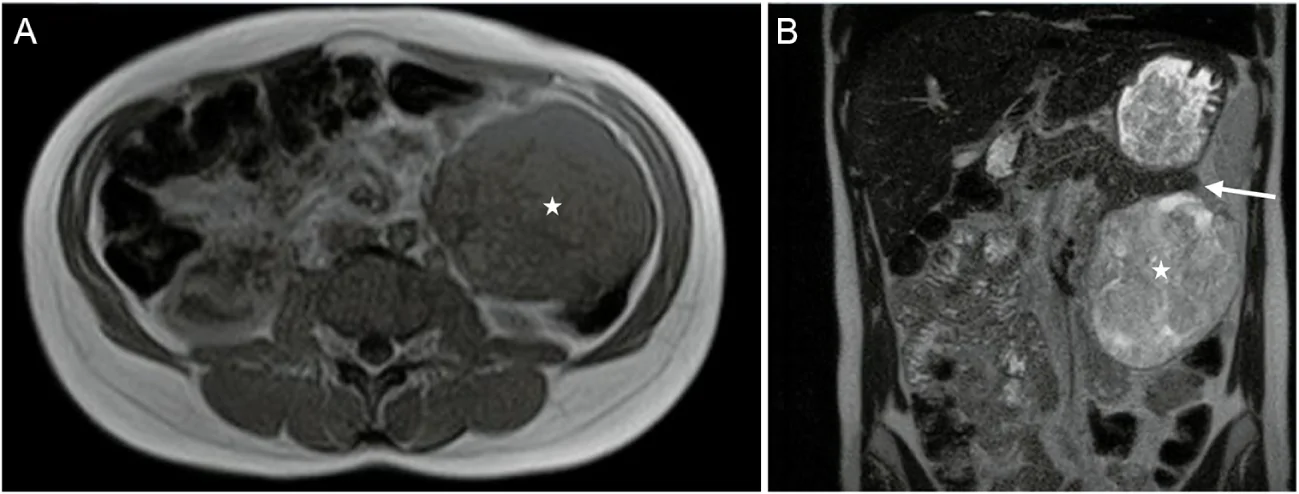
MRI of the abdomen – axial cut, T1 without contrast (A) and coronal cut, T2 with contrast (B) confirming the solid nature of the large lesion with necrotic areas (identified by a star) and dependence of the pancreatic tall (identified by an arrow).
